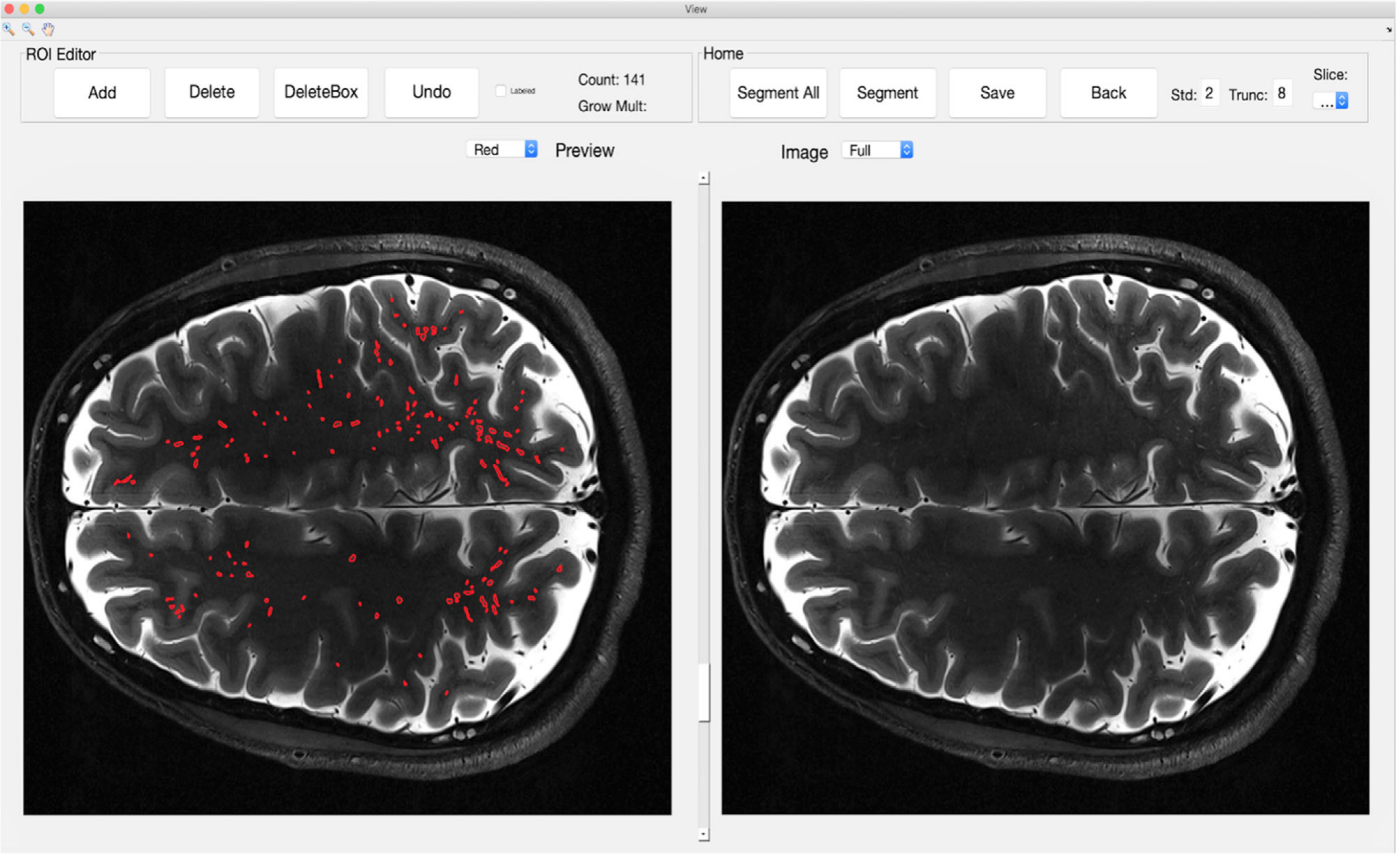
Unsegmented and segmented 7T MRI Scan. A side‐by‐side comparison of a segmented axial T2‐TSE slice (left) and the unsegmented image (right) in the PVS‐SAS program. Each red outlined object on the marked slice (left) delineates a PVS, as detected by the semi‐automated PVS marking, and confirmed by two manual readers
